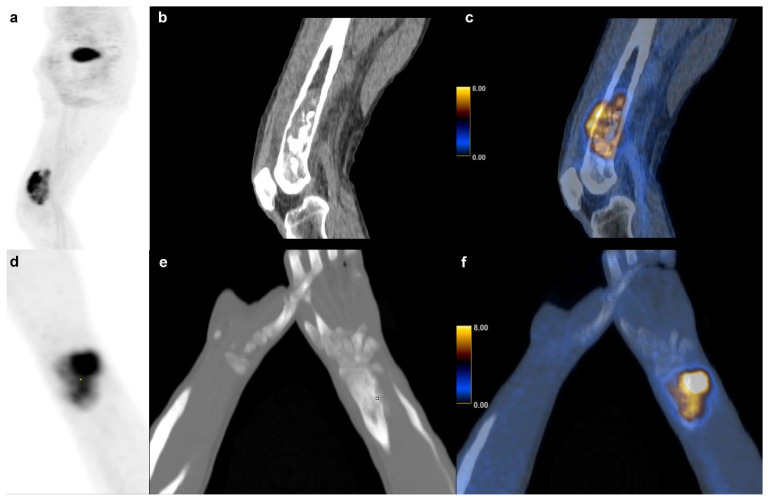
Bone sarcoma: Dedifferentiated chondrosarcoma of a 73 y.o. patient with a survival of 6 months (231.9 cm^3^) in the right distal femur (a) sagittal FDG PET maximum intensity projection (b) CT and (c) fused FDG PET/CT images. (d–f) Osteosarcoma in a 14 y.o. patient with a (60.1 cm^3^) of the right radius and a survival of at least 101 months.
